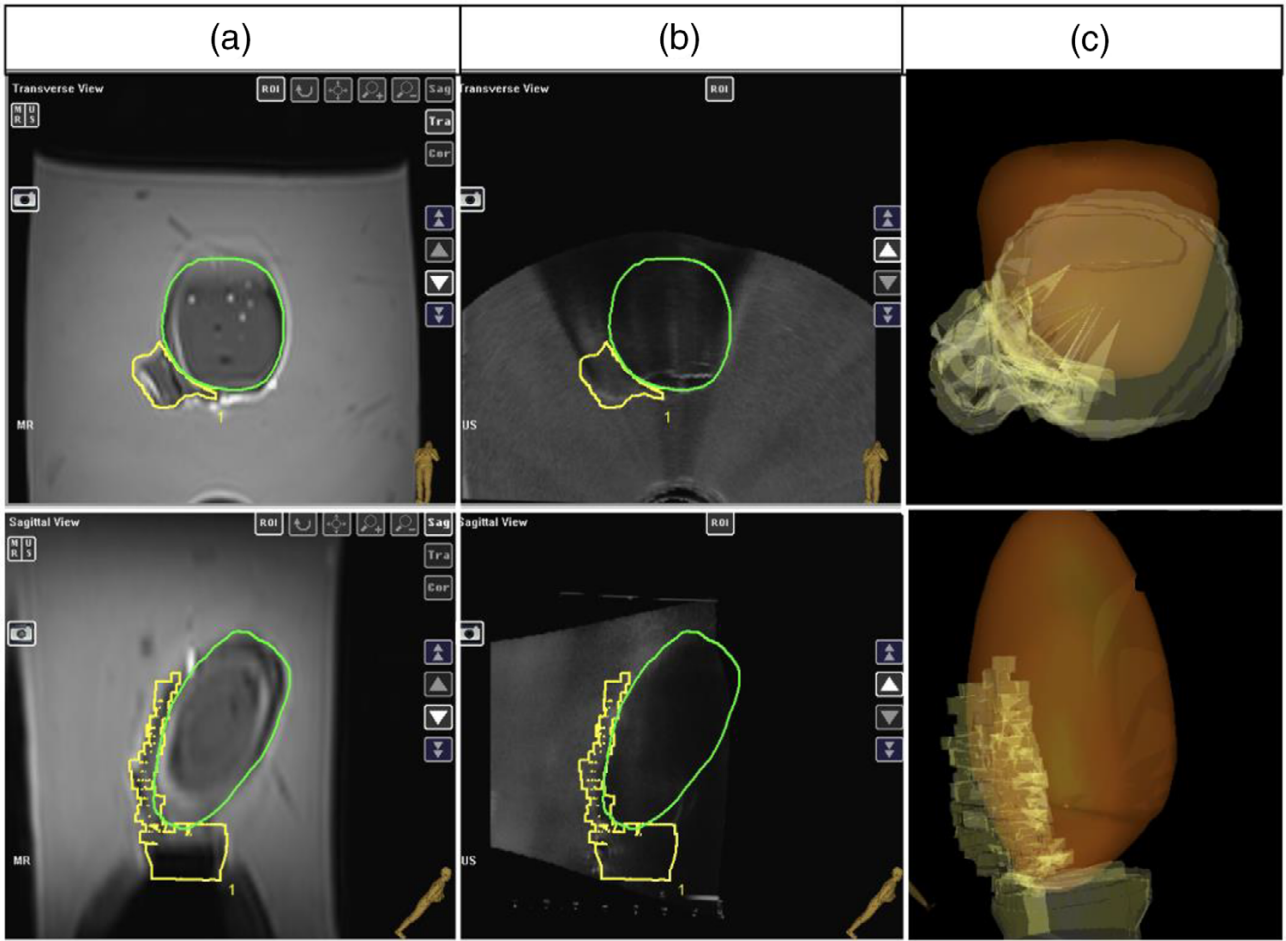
Transverse (top) and sagittal (bottom) views of contours used for targeting after T2w‐MR to TRUS registration. Post‐registration uterine (green) and CTV_HR_ (yellow) contour on MR (a) and TRUS (b); (c) 3D‐rendered model of the uterus (orange) and CTV_HR_ (yellow) for needle‐placement and targeting. TRUS, transrectal ultrasound.
